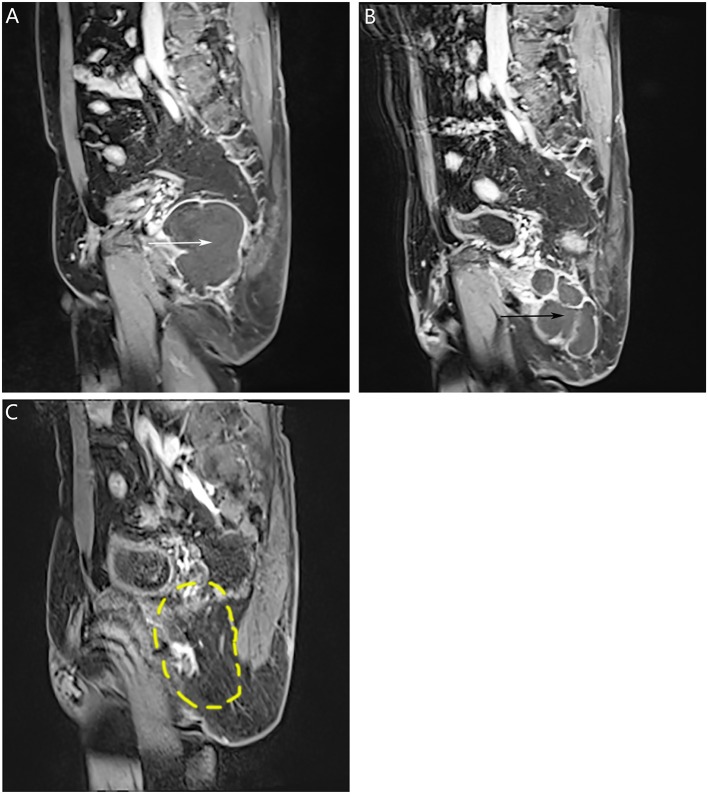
Pre-operative pelvic MRI. (A) Before first surgery in our hospital, contrast-enhanced pelvic MRI in sagittal view showed a 6.8 cm * 5.2 cm * 7.3 cm mass located at presacral space (white arrow). (B) Before second surgery in our hospital, contrast-enhanced pelvic MRI in sagittal view showed a 3.2 cm * 7.2 cm * 5.8 cm mass located at presacral space (black arrow). (C) One year after final surgery, enhanced pelvic MRI showed no signs of recurrence (the yellow dotted circle indicated the location of the previous mass).
